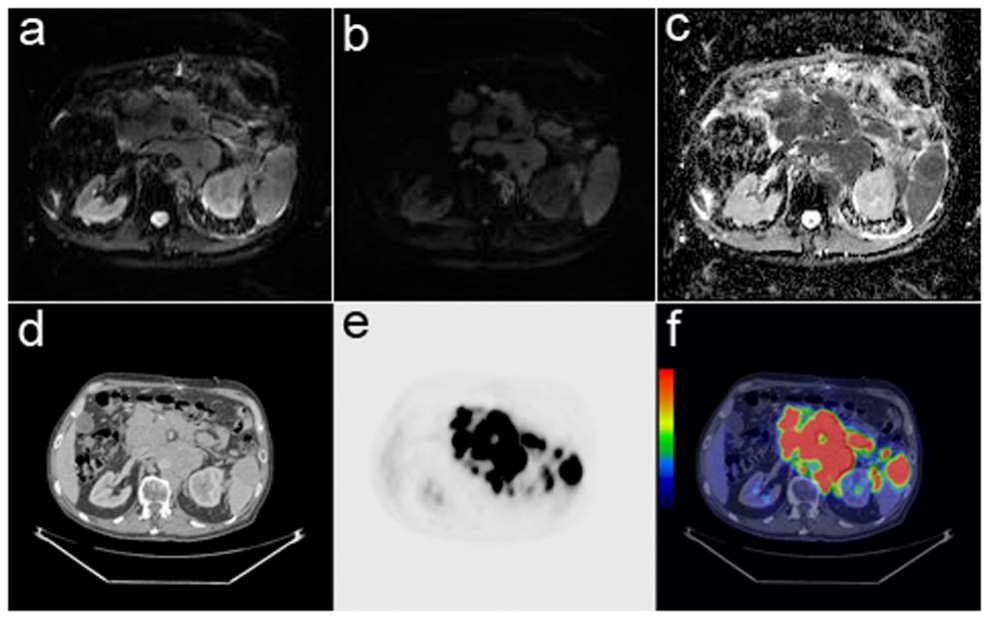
Diffusion-weighted MRI and PET/CT images showing the abdominal region tumor in a 76- year old male patient with diffuse large B-cell lymphoma. (a) B0 image. (b) Diffusion-weighted image with b value 800 s/mm^2^ showed the hyperintensity tumor, but it was not able to depict diffuse spleen involvement. (c) The corresponding ADC map showed the hypointensity tumor with ADC_min_ 0.34×10^−3^ mm^2^/s and ADC_mean_ 0.68×10^−3^ mm^2^/s. (d) Axial CT image. (e) FDG-PET image. (f) The fused PET/CT image showed the active tumor and spleen involvement with SUV_max_ 23.9.
